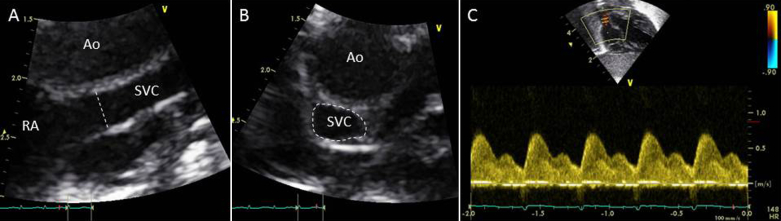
Echocardiographic quantification of superior vena caval flow, with (A) diameter measured from a modified parasternal long-axis view, (B) area measured from an axial view, and (C) velocity assessed from a low subcostal view. *Ao*, Aorta; *RA*, right atrium; *SVC*, superior vena cava.
